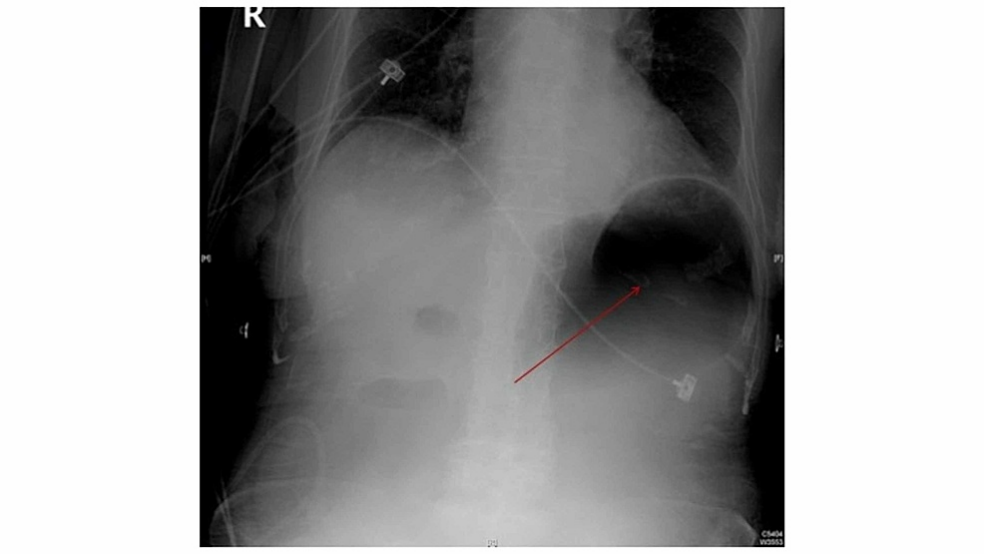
Abdominal radiograph revealed a distended stomach (red arrow) concerning for gastric outlet obstruction
